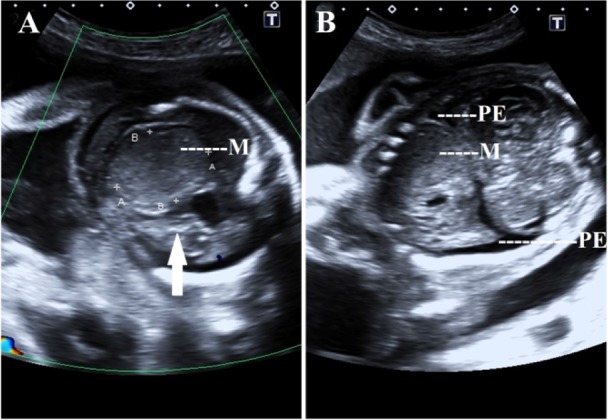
A 23 year old primi of tuberous sclerosis with fetal cardiac rhabdomyoma at 31 weeks of gestation resulting in IUFD. A: Color Doppler ultrasound showing absent cardiac activity (arrow), note the mass (M) and subcutaneous edema. B: Ultrasound in coronal section showing the mass (M) arising from the left ventricle, note the bilateral pleural effusion (PE)
